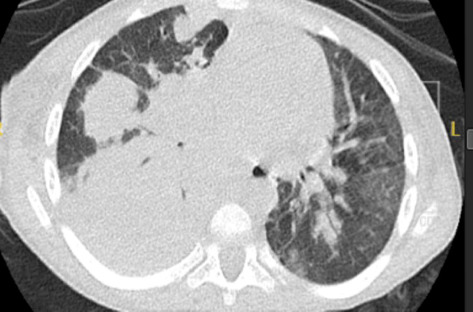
Chest CT showing severe necrotizing pneumonia and pneumatocele formation.
